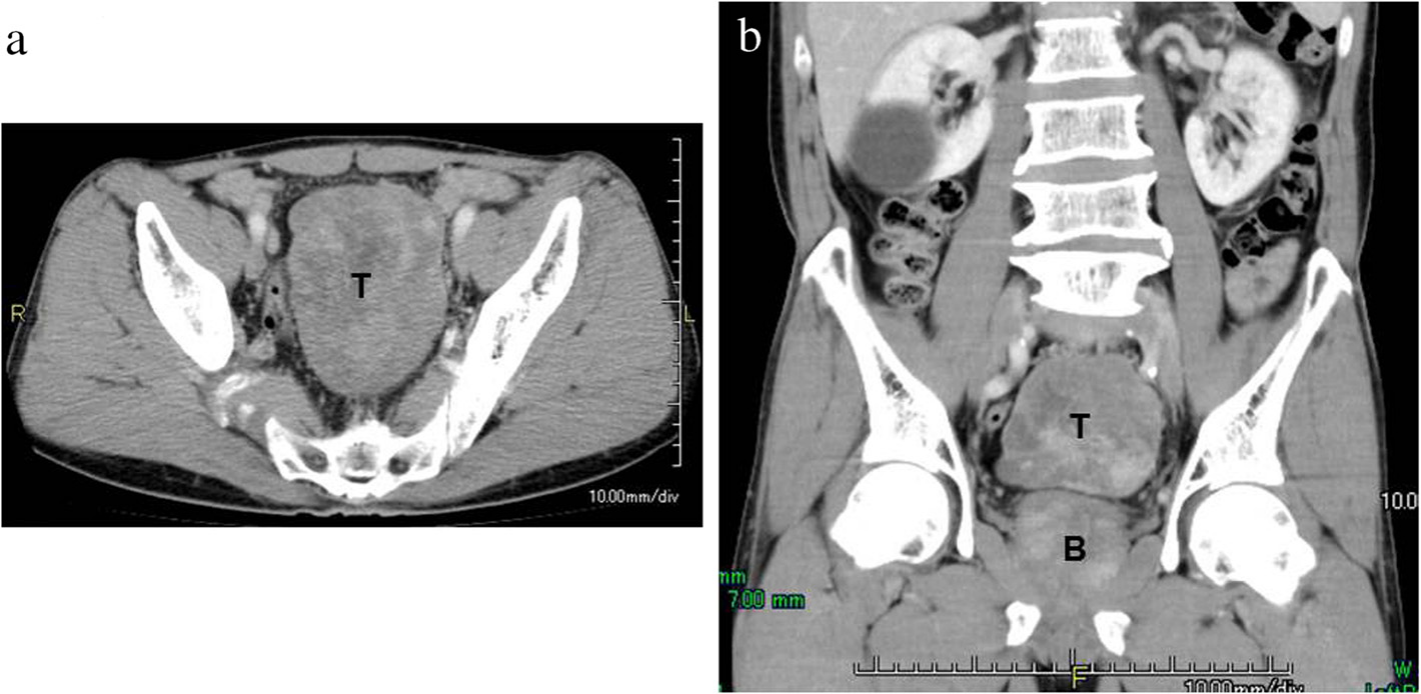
CT showed a 10 × 8 × 7-cm, heterogeneously CE pelvic tumor that was slightly lobulated and included hypervascular regions. (a) Transverse view. (b) Coronal view. T: tumor, B: bladder.
